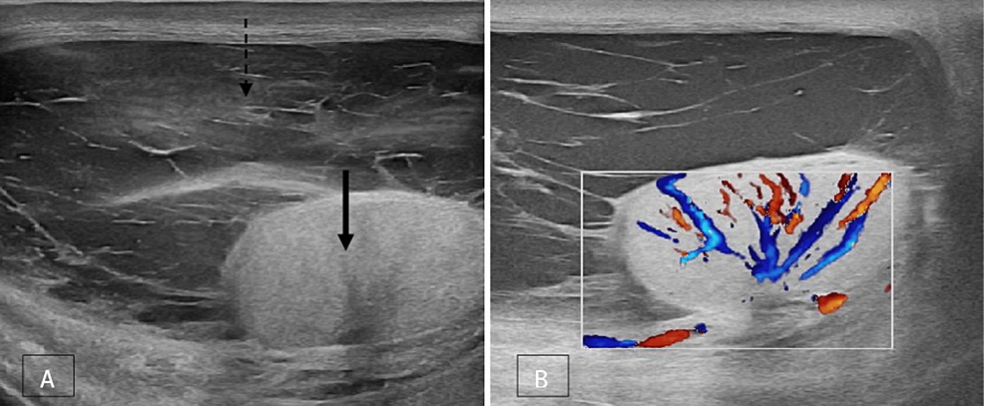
Acute orchitis with pyocele A, B Grey-scale and colour Doppler images of the right testis (solid black arrow) shows a heterogeneous echotexture with increased vascularity and pyocele (dotted black arrow).
